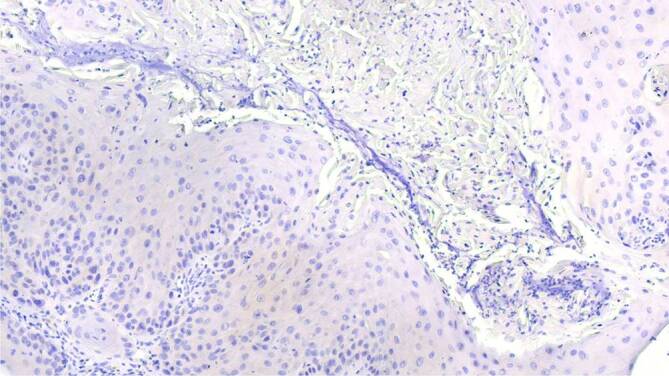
Zur weiteren operativen Sanierung der Zungenläsionen wurde der Patient in eine Klinik für Mund‑, Kiefer- und Gesichtschirurgie verlegt. Die präoperative Ausbreitungsdiagnostik inklusive Computertomographie von Hals und Thorax, Sonographie des Abdomens und Ösophagogastroduodenoskopie ergab keine pathologischen Befunde. Es erfolgte eine Zungenteilresektion links mit intraoperativer Schnellschnittdiagnostik, die nun ein 1,3 cm tief in die Muskulatur infiltrierend wachsendes Plattenepithelkarzinom mit Lymphangiosis carcinomatosa, aber ohne Gefäßeinbrüche oder perineurale Tumorausbreitung zeigte. Die Defektrekonstruktion erfolgte mittels eines mikrovaskulär reanastomosierten, perforatorgestielten, rein kutanen Hauttransplantates vom rechten Oberschenkel (Abb. 5). Zudem wurde eine Neck-Dissection durchgeführt, die keinen weiteren Tumorbefall zeigte. Unter Berücksichtigung der gesamten Befunde handelte es sich somit um ein Zungenkarzinom auf dem Boden eines Lichen planus mucosae mit der Tumorklassifikation pT3, G2, pN0, L1, V0, Pn0, R0. Nach kompletter Einheilung des Transplantates erfolgte eine adjuvante Radiochemotherapie (60 Gy-Strahlentherapie und kombinierte Chemotherapie mit Cisplatin und 5‑Fluoruracil); 24 Monate nach Radiochemotherapie war der Patient weiterhin tumorfrei. Aufgrund der durch den genitalen Befall des Lichen planus entstandenen Synechienbildung im Bereich des Penis erfolgte eine Zirkumzision, die komplikationslos verlief.
